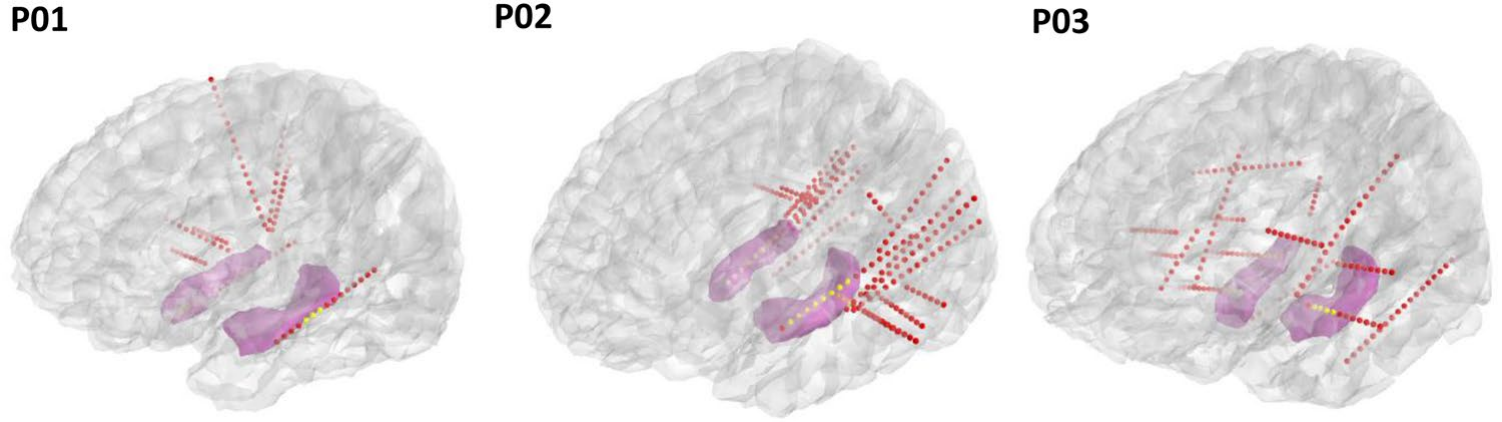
sEEG implantation locations. Electrodes inserted using stereotactically guided implantation. Electrode locations superimposed over the patient’s reconstructed brain from MRI.
